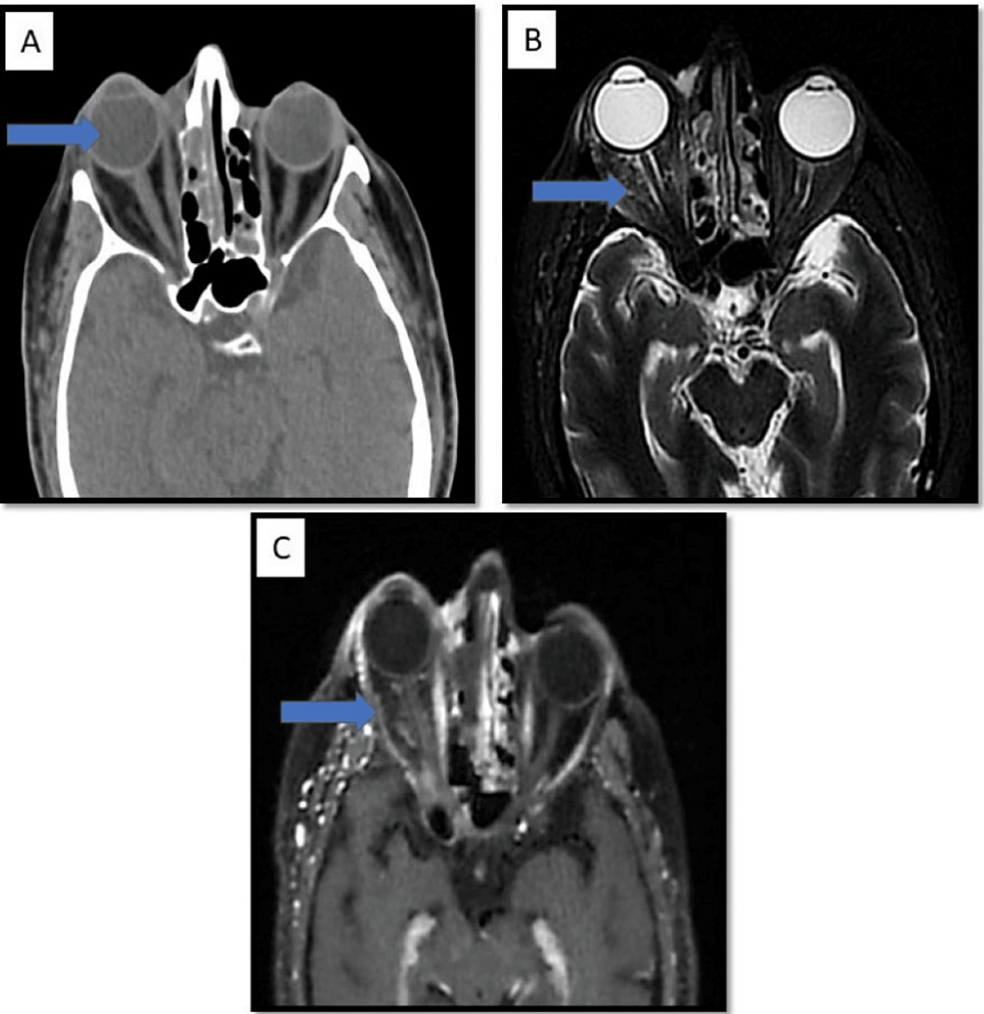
Orbital involvement: case 2 Image A: an axial CT image, shows proptosis of the right eye globe. Image B: T2 Stir-axial MR image shows fat stranding noted involving the intraconal orbital fat. Image C: Enhancement of medial rectus muscle and pre- and post-septal compartments of the right orbit on a post-contrast study
